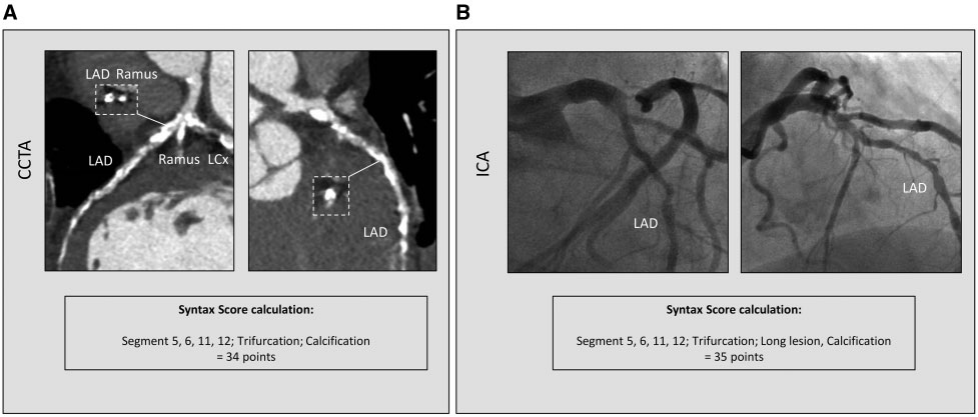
Calcified coronary lesion without Syntax score discrepancy. A case of a heavily calcified lesion not affecting the calculation of CCTA-derived (A) and ICA-derived (B) SYNTAX scores. CCTA: coronary computed tomography angiography; ICA: invasive coronary angiography; LAD: left anterior descending; LCX: left circumflex.
